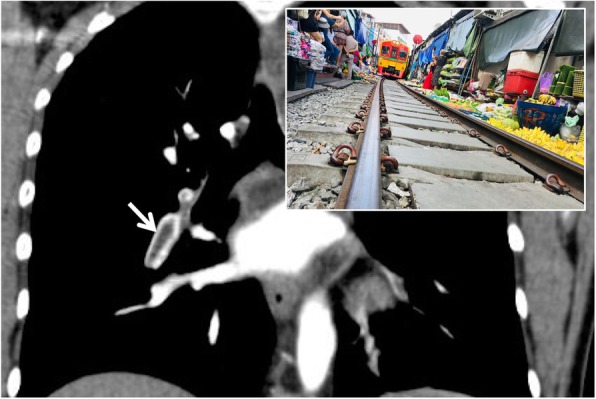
Railway track sign. The coronal CT image well depicts linear and centric filling defect (white arrow) inside the lumen of an arterial lung vessel—due to acute embolism; the contrast, surrounding the central endoluminal defect of opacification, reproduces a railway track appearance. The railway track sign is very similar to the polo mint sign, which instead describes the thrombosed vessel displayed according to an axial plane
